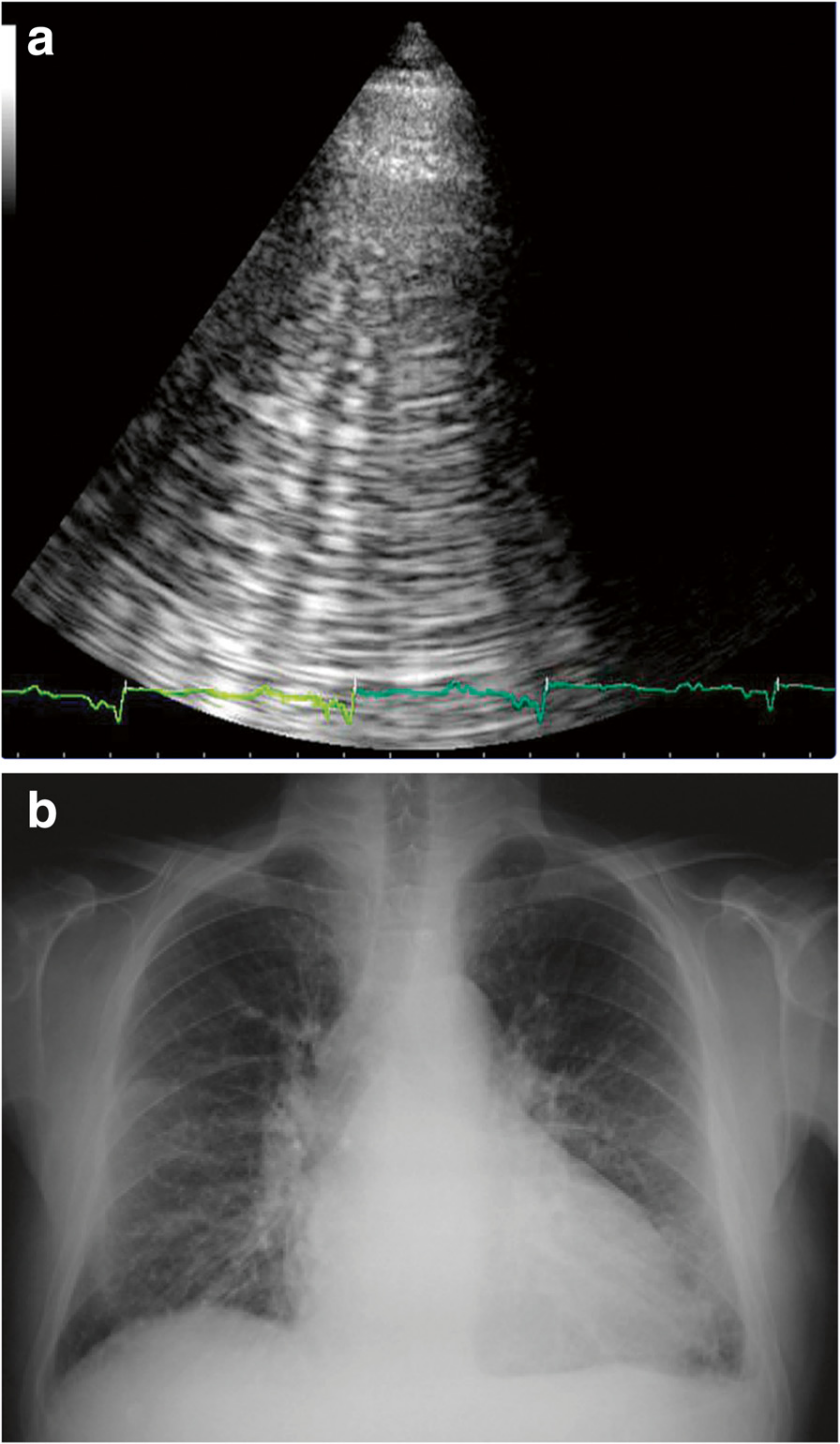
Images of B-lines from a lung ultrasound and b chest X-ray scan suggestive of AHF diagnosis. Reprinted from [23, 71]
